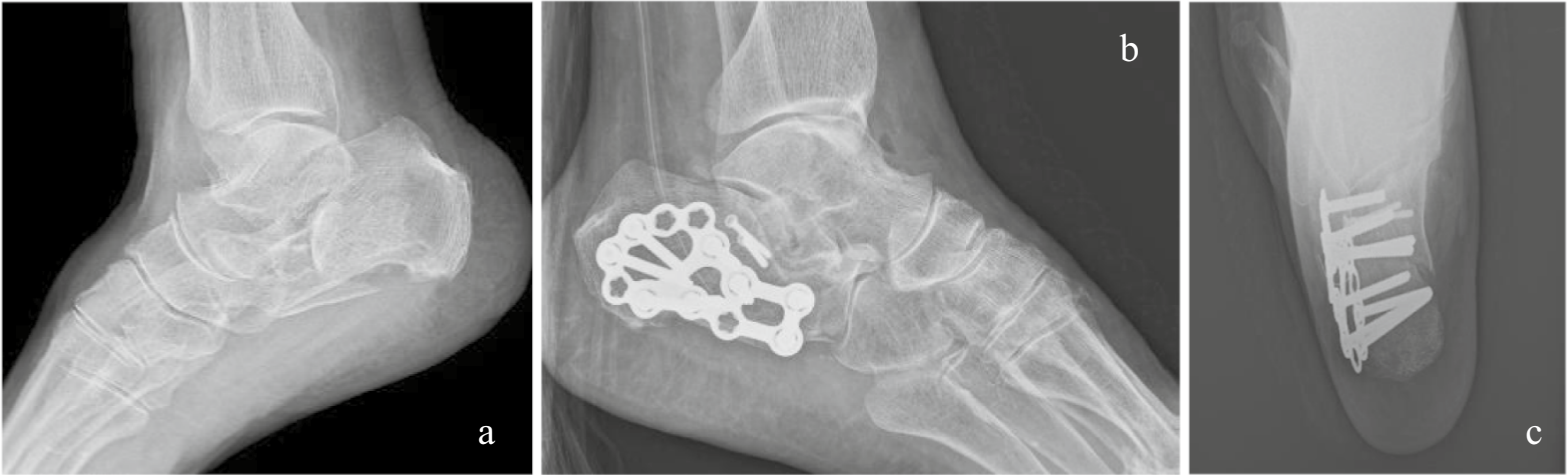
a Radiograph of a comminuted fracture of the calcaneus. b Lateral view and c axial view Harris of postoperative fracture of the calcaneus. Reduction and synthesis performed with lateral plate and screws and sustentaculum tali screw
